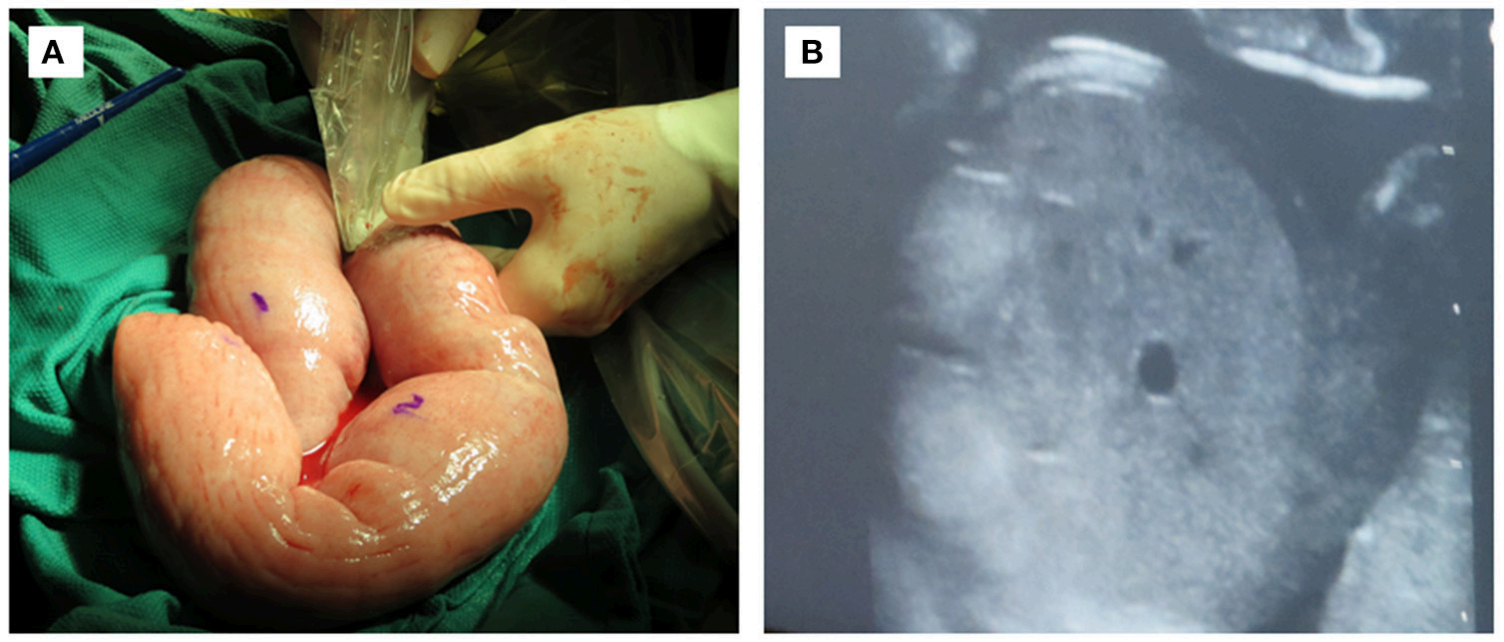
In utero* injection of fetal intraperitoneal space via laparotomy. (A) Exteriorized uterus at 40 days gestation being ultra-sounded for fetuses. Water soluble marker can be used for marking fetuses. (B) Ultrasound image of fetal liver with which human CD34^+^ stem cells would be injected.
